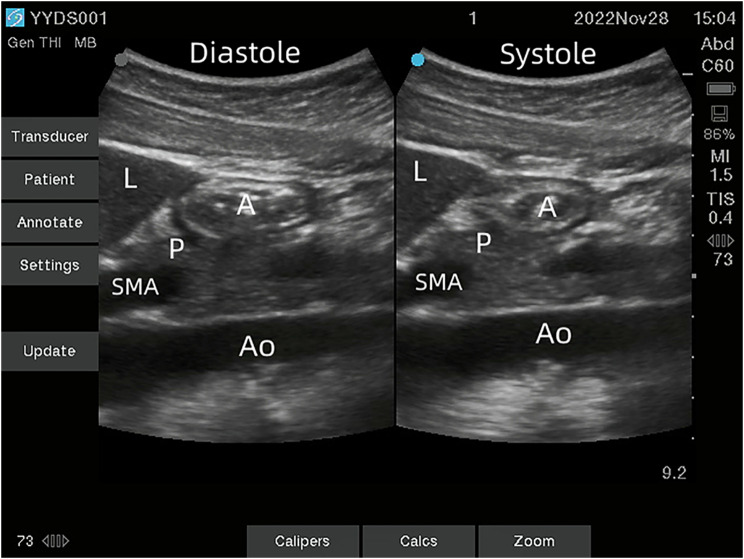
Diastolic and systolic ultrasound images of the gastric antrum after drinking. L: liver; A: gastric antrum; P: pancreas; SMA: superior mesenteric artery; Ao: abdominal aorta
